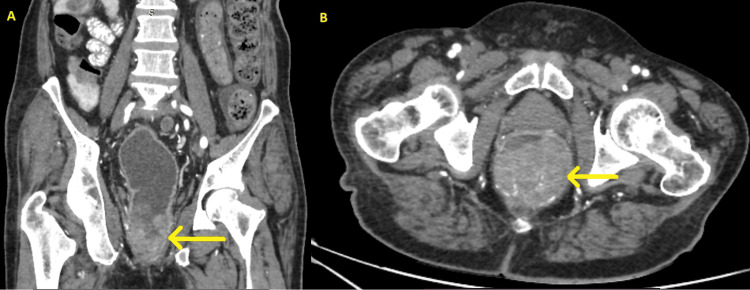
CT images of the lesion in (A) coronal and (B) axial sections.
